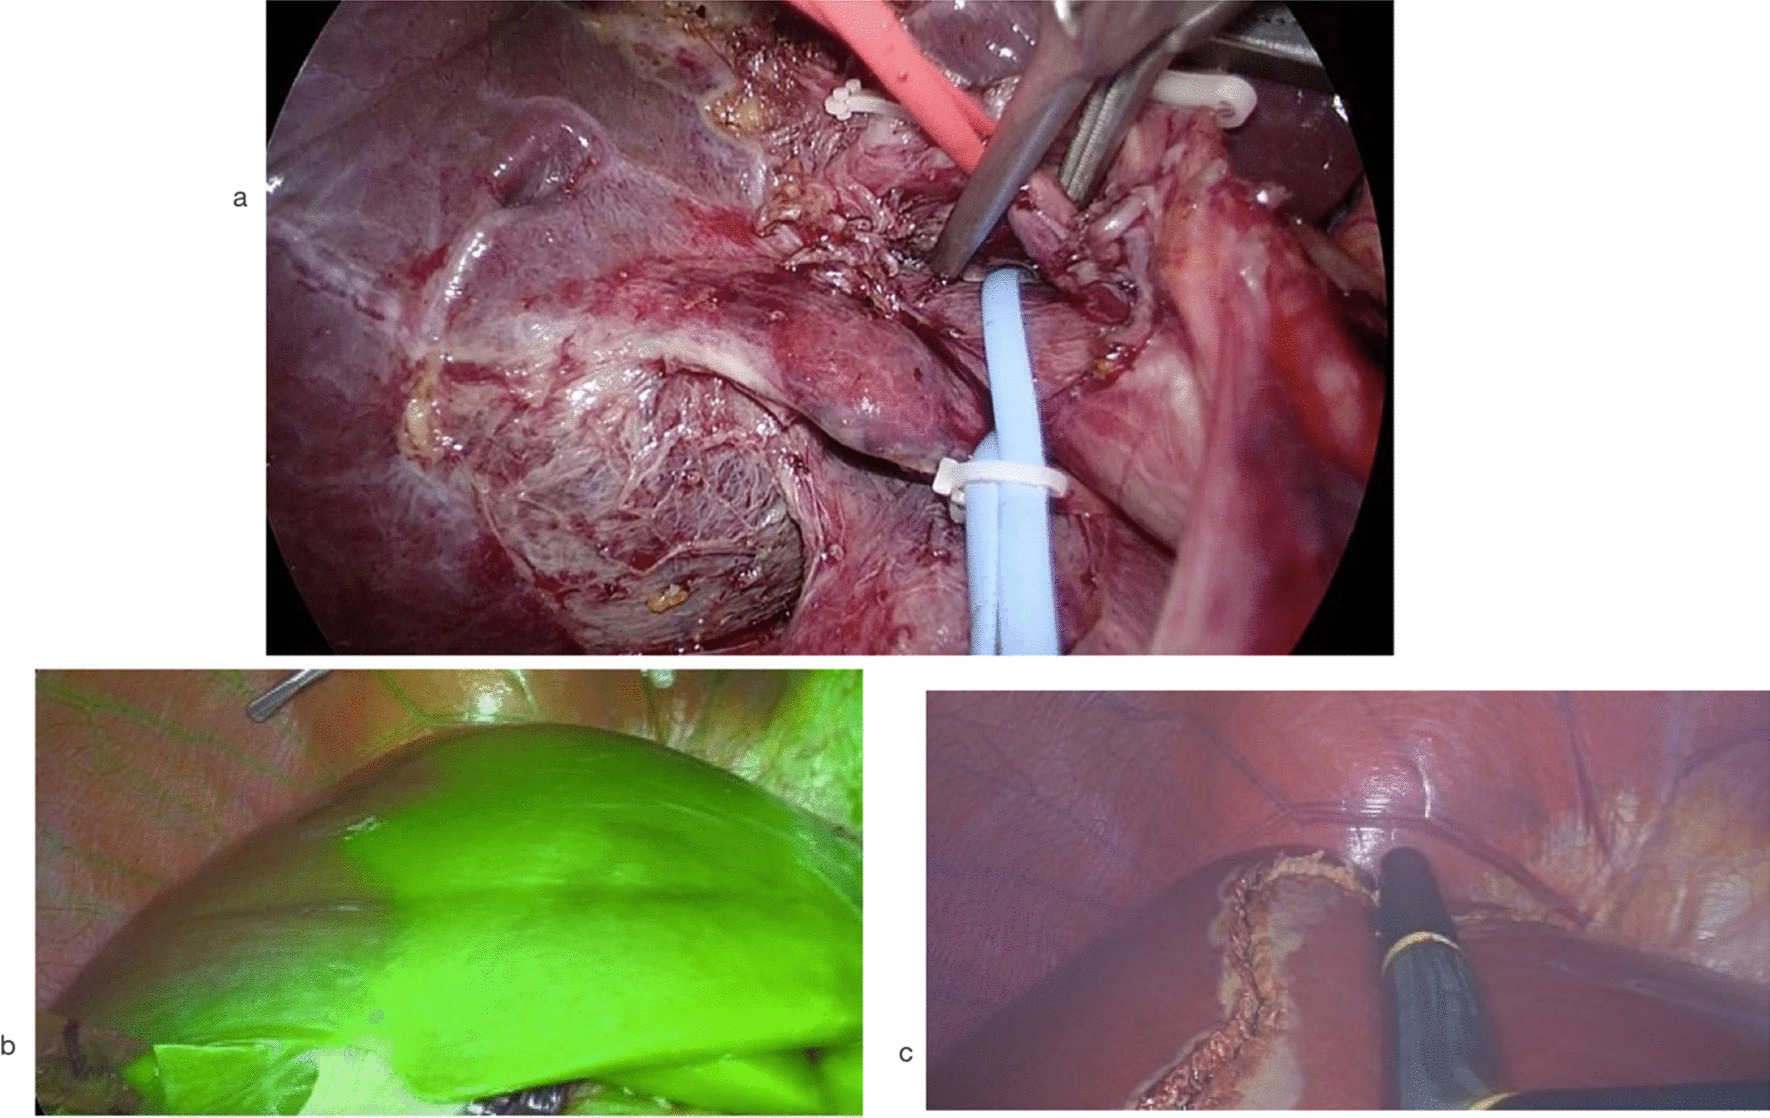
Vascular inflow identification and ischemic demarcation. a The right hepatic artery and right portal vein were wrapped with red and blue elastic tape, respectively, and then temporarily controlled with an endo-bulldog clamp. b Ischemic demarcation line demonstrated and marked by overlaid near-infrared imaging. c Laparoscopic ultrasound was used to identify the middle hepatic vein
